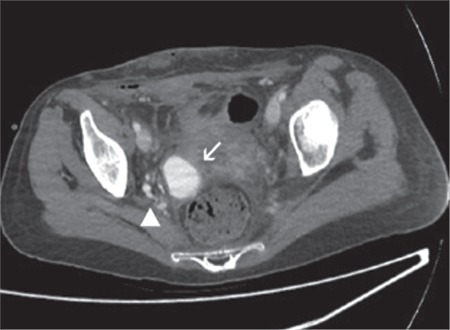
Computed tomography images acquired in the arterial phase show enlarged vascular structured connected to the arteriovenous fistula (arrow head) and aneurysm sac (white arrow)
